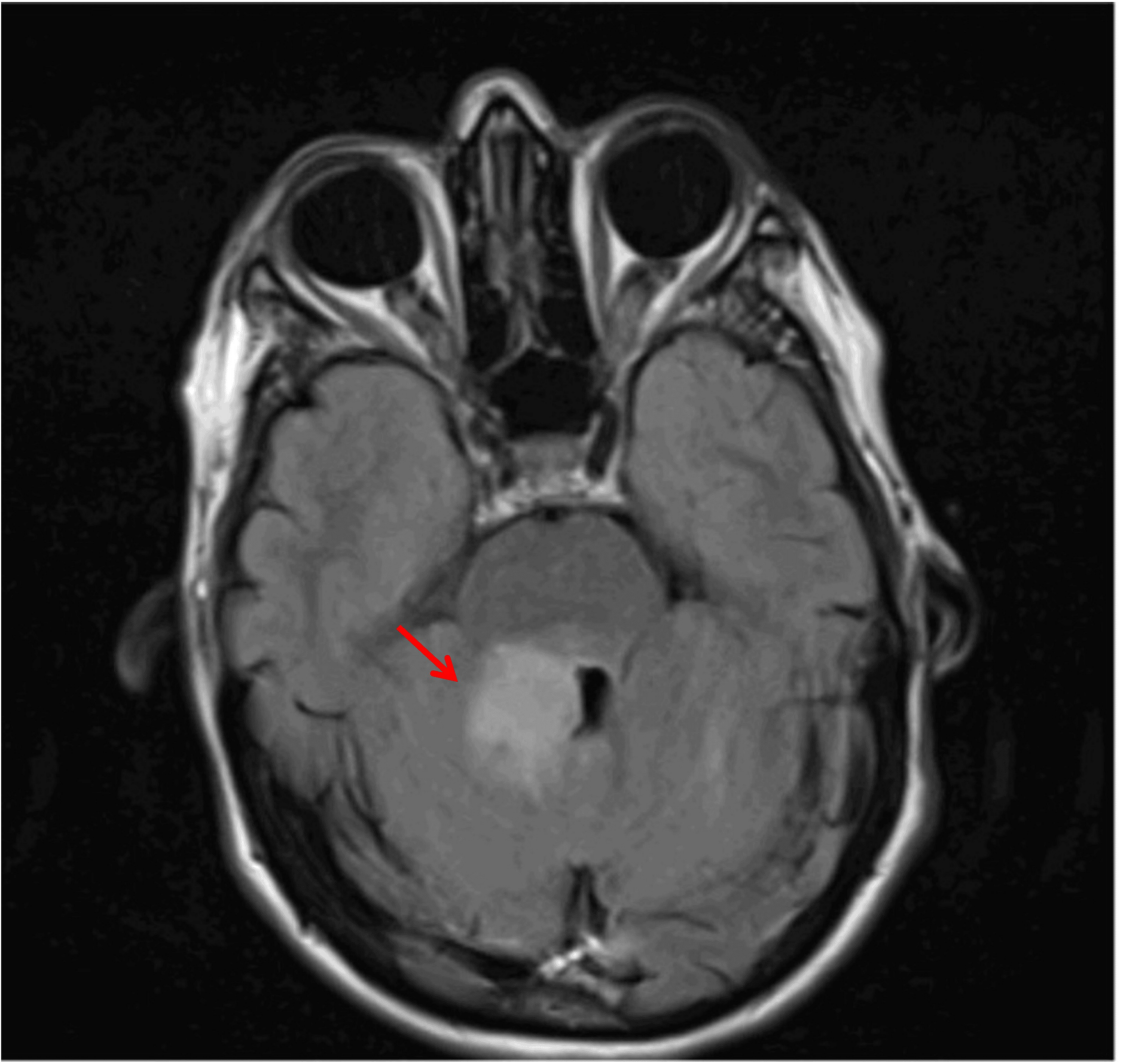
Brain magnetic resonance imaging (T2-FLAIR sequence) showing an expansile lesion (red arrow) of the right cerebellar peduncle with posterior fossa involvement. FLAIR: fluid-attenuated inversion recovery.
